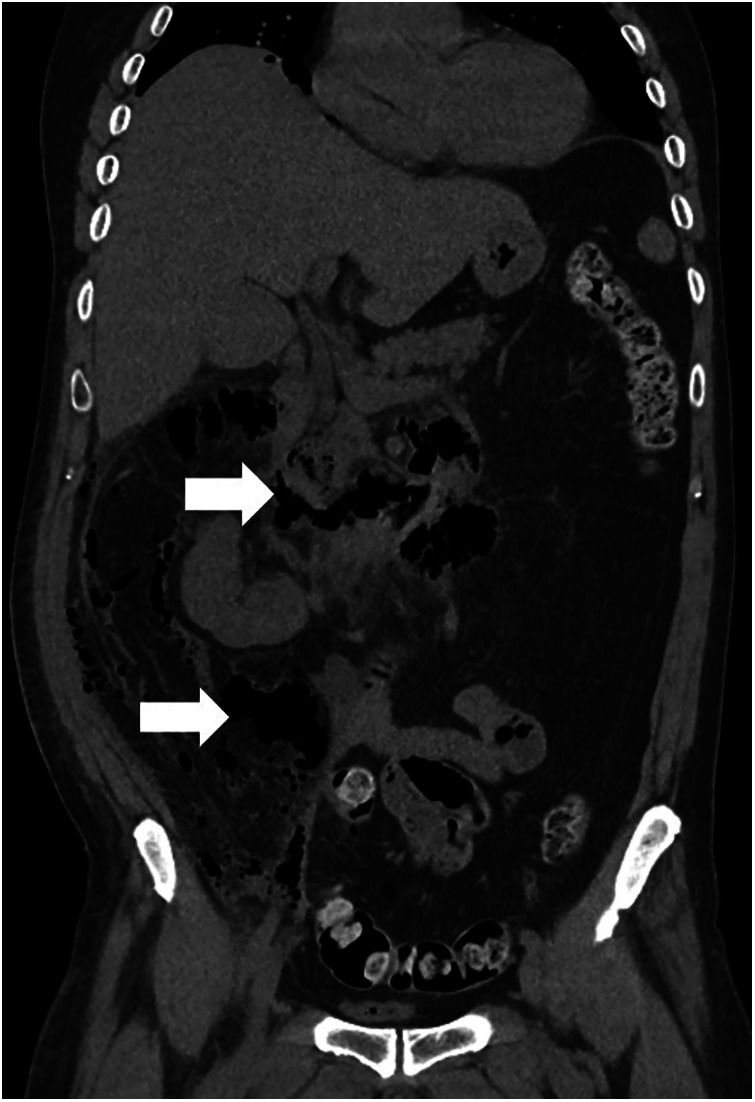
Noncontrast abdominal CT demonstrating extensive retroperitoneal free air (arrow) consistent with retroperitoneal extension of infection from necrotizing fasciitis (Fournier's gangrene).
